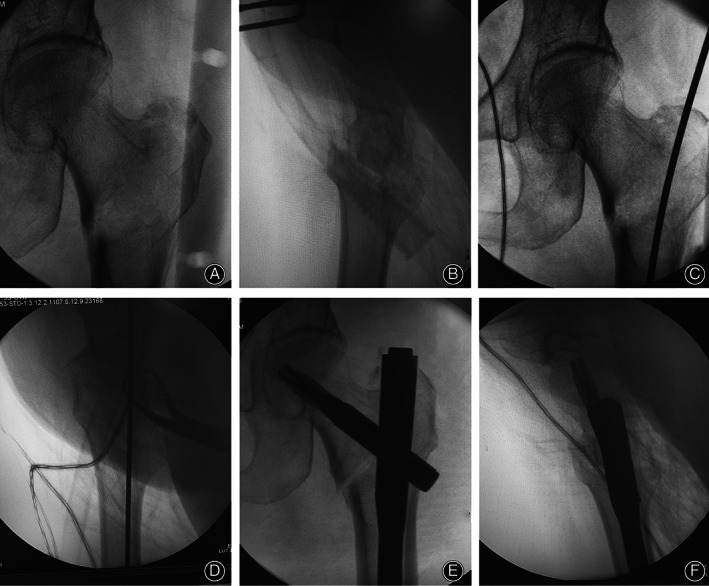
Intraoperative fluoroscopy images. Anteroposterior (A) and lateral (B) radiographic images of closed reduction facilitated by double reverse traction repositor. Quality of reduction is accepted as “excellent.” Anteroposterior (C) and lateral (D) radiographic images of the guide wire insertion. Anteroposterior (E) and lateral (F) radiographic images of the PFNA‐II nailing demonstrated that the anatomic reduction was maintained by the double reverse traction repositor and that the fixation quality was optimal.
